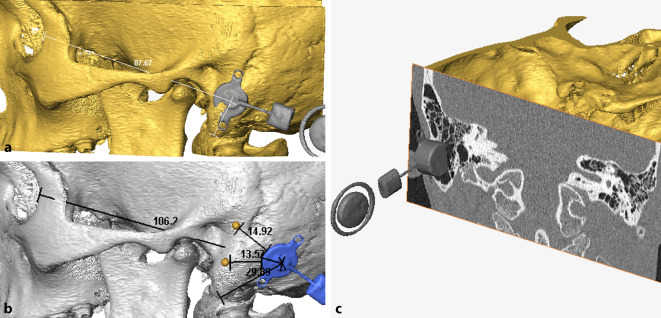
Preoperative planning, demonstrated as an example in patient 1. a Optimal placement of the floating mass transducer (FMT) without planning of prosthesis anchors (sinodural angle). b 3D planning with prosthesis anchors and retrosigmoidal FMT implant position with marked distances to landmarks and prosthesis anchors for intraoperative transfer. c View of the initially planned sinodural angle position with coronal computed tomography scan
